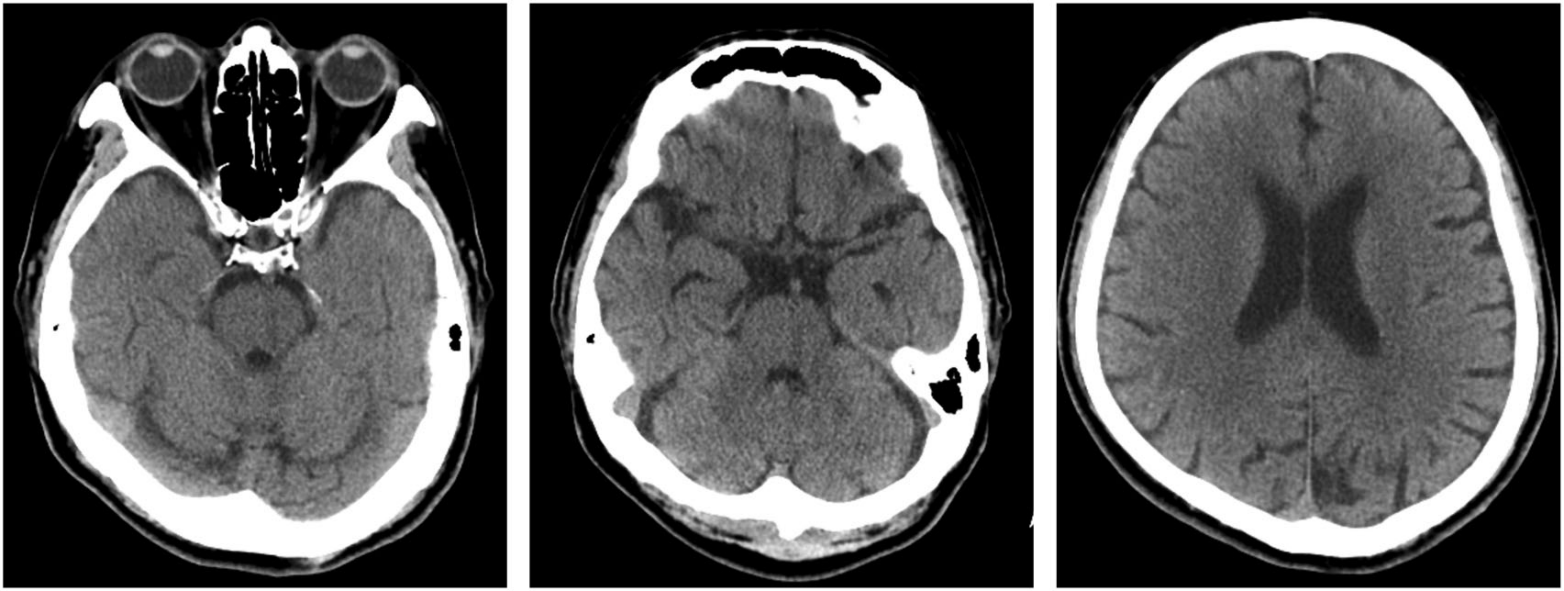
Non-enhanced computed tomography (CT) of the brain showed no established major vessel vascular territory infarct.
